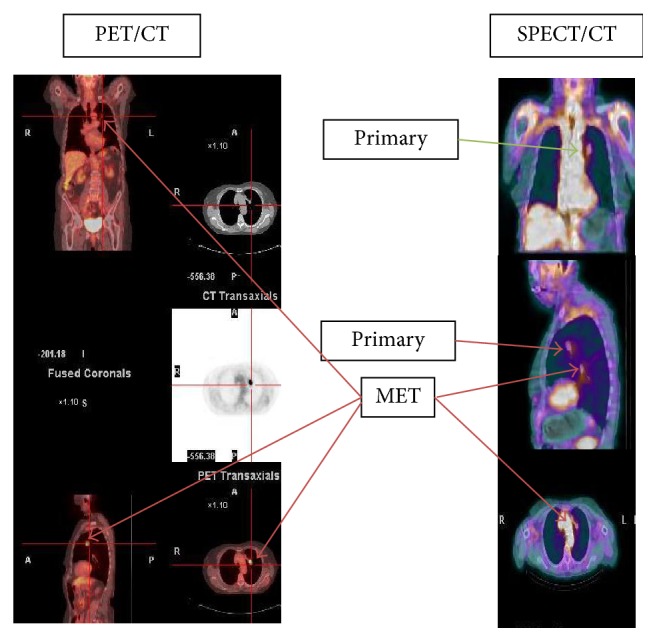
Comparative SPECT/CT: ^99m^Tc- EC-G and PET/CT: ^18^F- FDG image sets on a 61-year-old patient having a biopsy confirmed primary NSCLC and diagnostic CT contrast confirmed metastasis to the lung.
